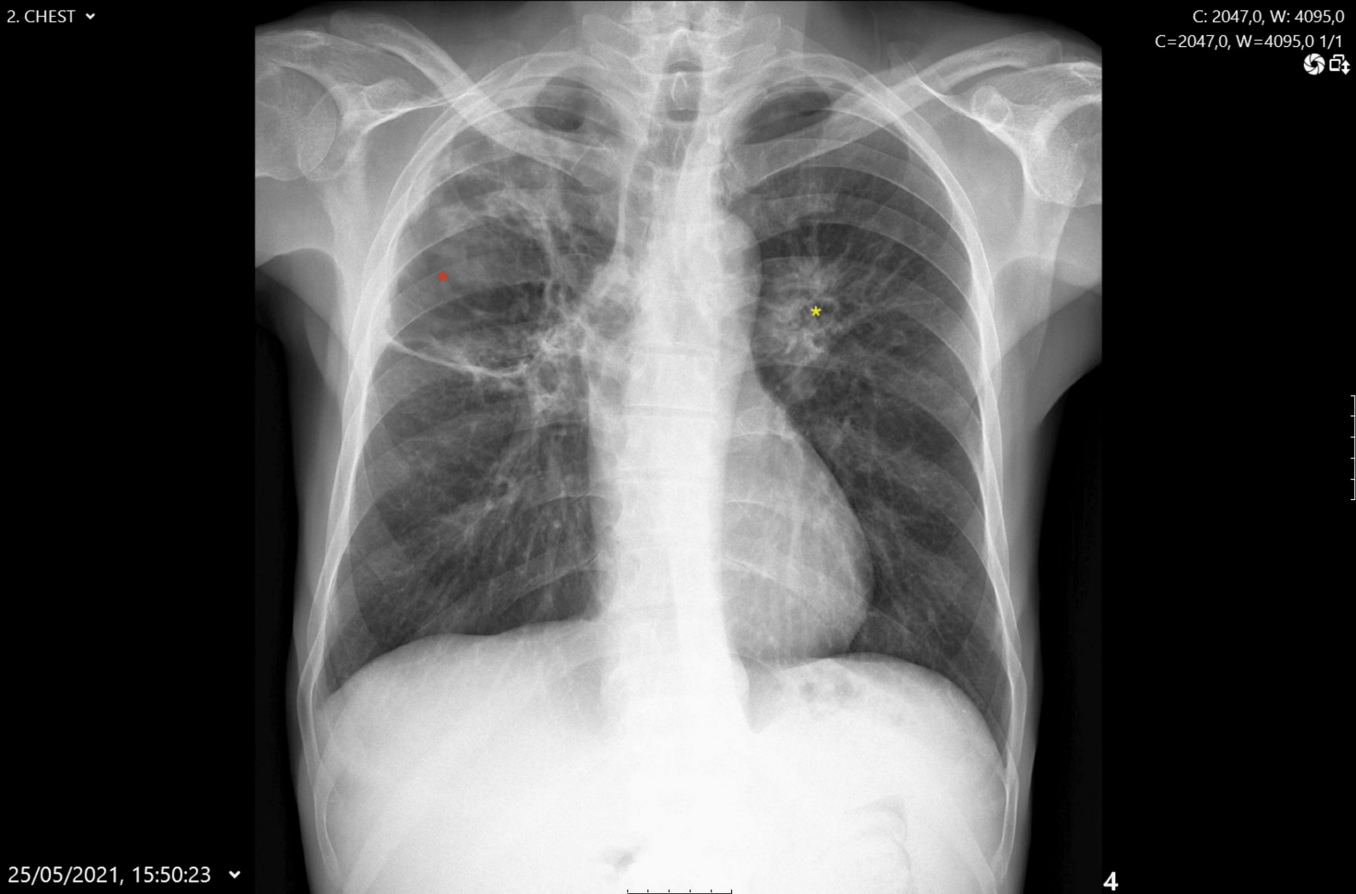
Chest radiograph following initiation of immunosuppressive therapy Post-immunosuppressive therapy chest radiographs taken on May 25, 2021, in anteroposterior view. The images show a reduction in the thickness of the cavitary wall, particularly in the right upper lobe (red asterisk) and left lung (yellow asterisk), with the cavitations still present. These findings suggest a reduction in the inflammatory activity following treatment with corticosteroids and rituximab, consistent with granulomatosis with polyangiitis (GPA)
